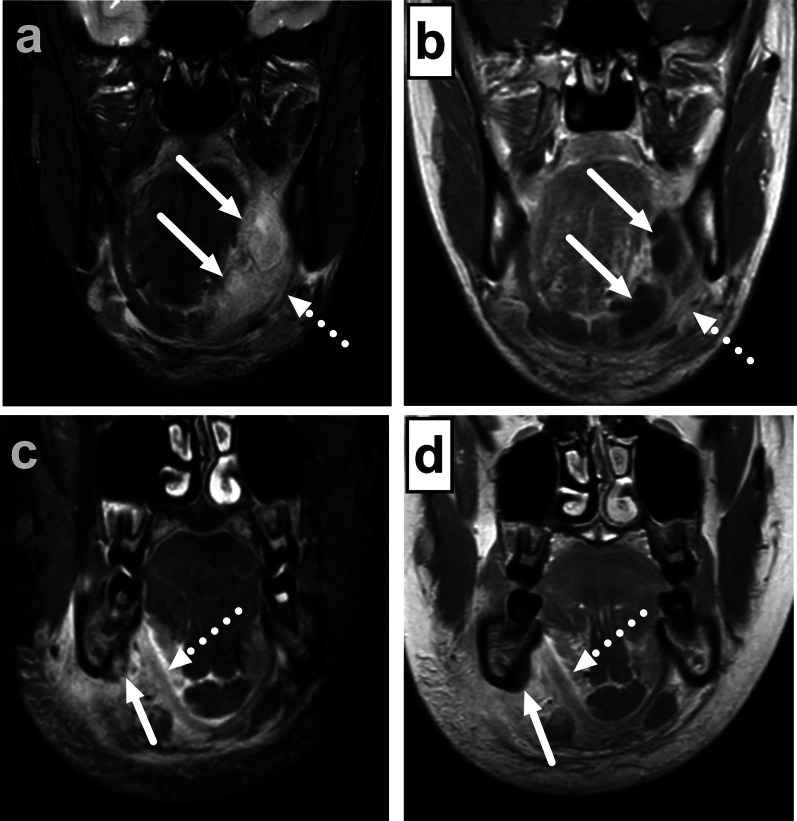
Odontogenic maxillofacial infections in two patients (a and b, c and d). Coronal fat-suppressed T2-weighted (a, c) and post-contrast T1-weighted (b, d) images show sublingual abscesses (arrows in a and b) above the mylohyoid muscle (dotted arrow), and a submandibular space subperiosteal abscess (arrow in c and d) below the mylohyoid muscle (dotted arrow). Image modified from ref. [11]
